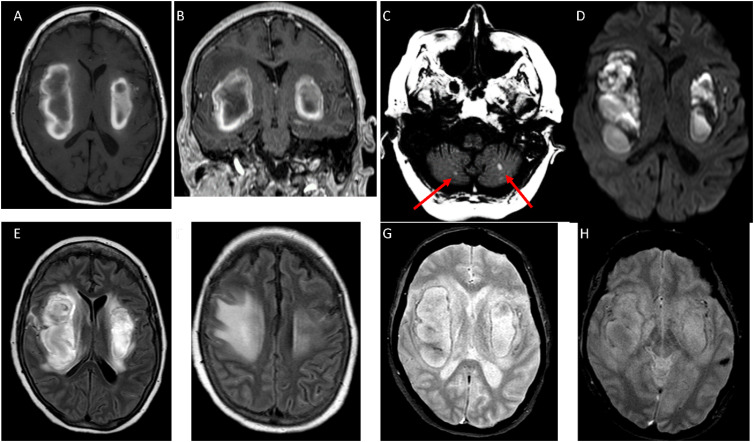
(A) Axial T1 spin eco (SE) showed central hypointense with peripheral hyperintense lesions on bilateral deep white matter with effacement of the lateral and third ventricles. (B) Coronal reconstruction of a volumetric T1 gradient-echo (GRE) showed central hypointense with peripheral hyperintense lesions on deep white matter. (C) Axial T1 SE showed two small hemorrhages on both cerebellar hemispheres (red arrows). (D) Diffusion-weighted imaging revealed possible restriction on bilateral deep white matter compatible with subacute hemorrhages. (E): Axial FLAIR at basal ganglia level showed hyperintensity lesions on bilateral deep white matter with surrounding edema. (F) Axial FLAIR at supraganglionic level showed extensive bilateral edema. (G): Axial T2* at basal ganglia level showed hyperintensity lesions on deep white matter with hypointensity due to subacute hemorrhages. (H) Axial T2* at lower basal ganglia level showed hyperintense lesions on bilateral deep white matter with hypointensity with blooming effect due to subacute hemorrhages and there was another small hemorrhage on the left temporal lobe.
